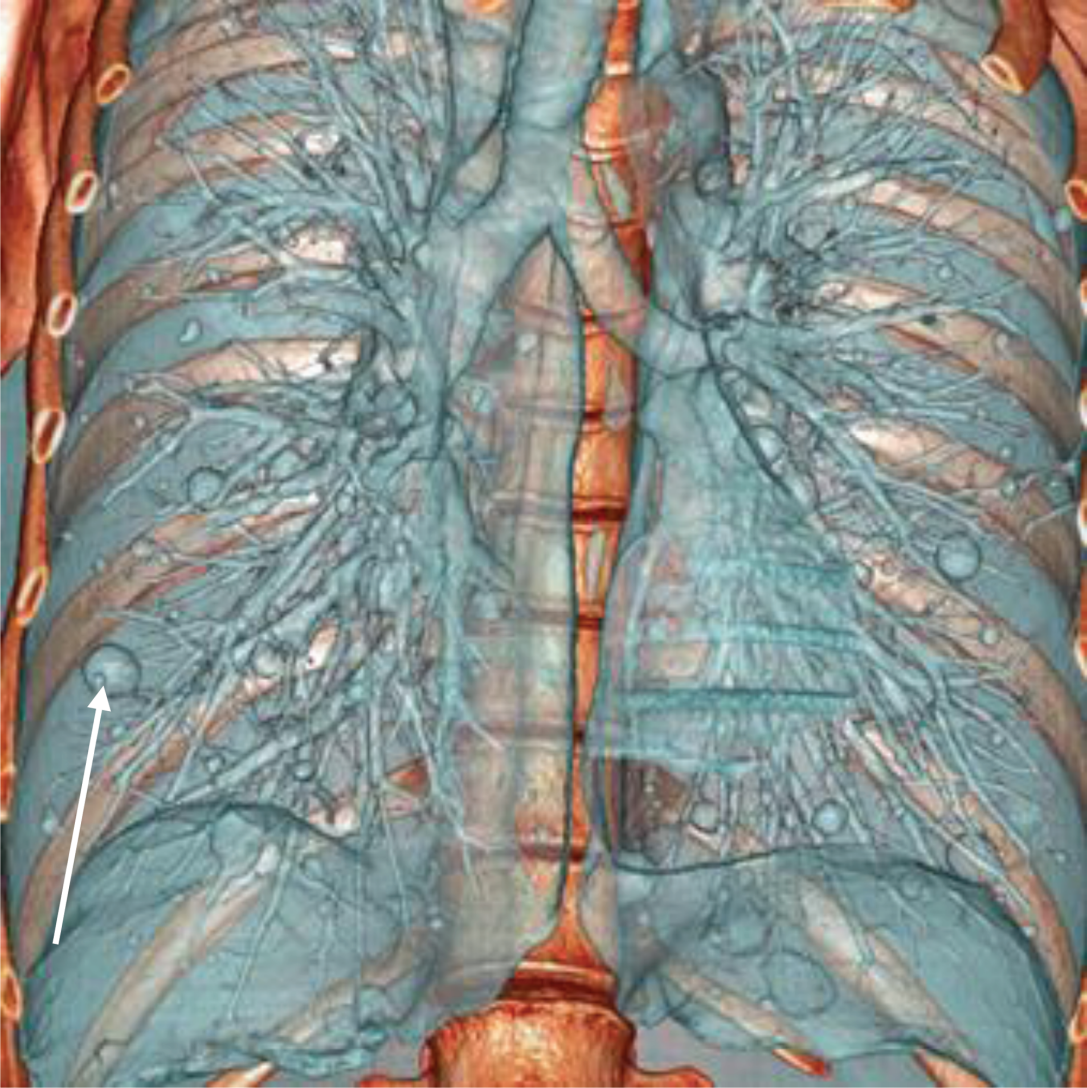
3D reconstruction of a chest CT scan, anterior view. Lung nodules in a snowfall pattern in the context of pulmonary benign metastasizing leiomyomatosis in Patient 2. The patient was started on goserelin, 3.6 mg subcutaneous every 28 days. The disease has remained stable, with the lung nodules reaching no more than 13 mm in diameter. The white arrow is pointing to one of the nodules, in the right lung.
